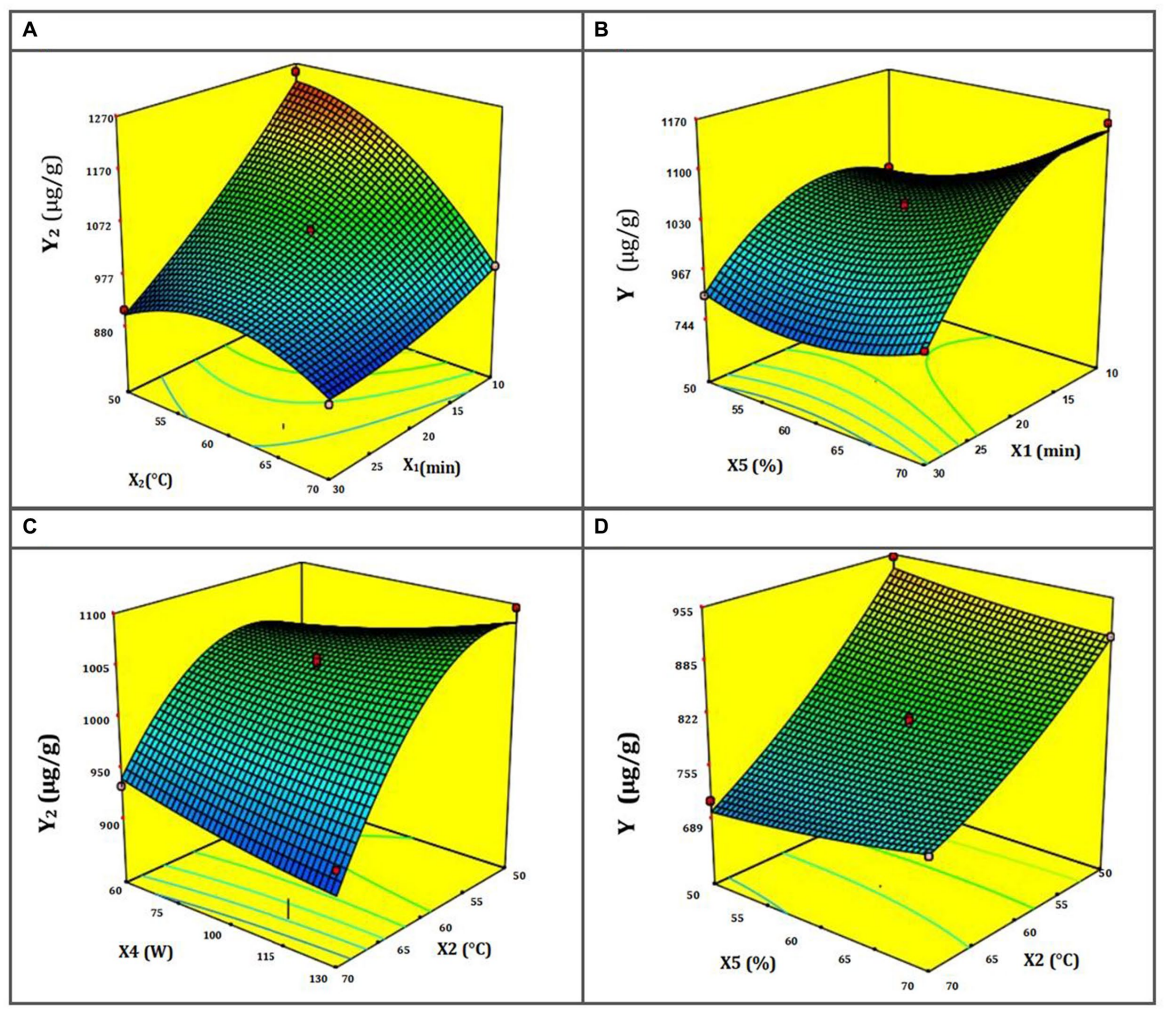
(A–D) Response surface for NA DES-UAE from ST as a function of the temperature and duration of ultrasound (A); the power and duration of ultrasound (B); the power and temperature of ultrasound (C); and the water content in DES-1 and the temperature of ultrasound (D).
